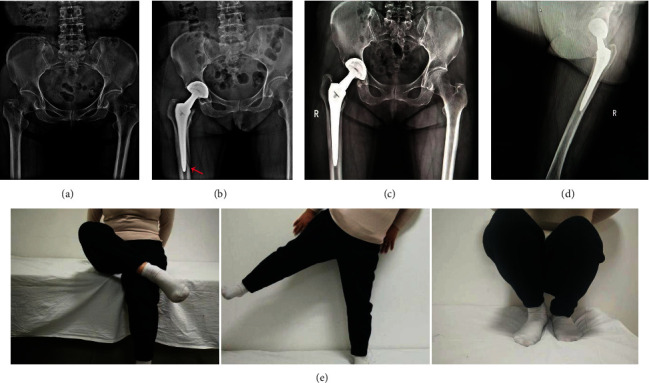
(a) Preoperative X-ray of a 59-year-old female patient with right femoral neck fracture. (b) The results of X-ray showed that intraoperative periprosthetic fracture occurred (red arrow). (c) The results of X-ray 3 months after surgery showed that the reconstruction of the leg length and offset of hip joint was good. (d) X-ray at 6 months after surgery. (e) The pictures showed good function and stable hip joint.
